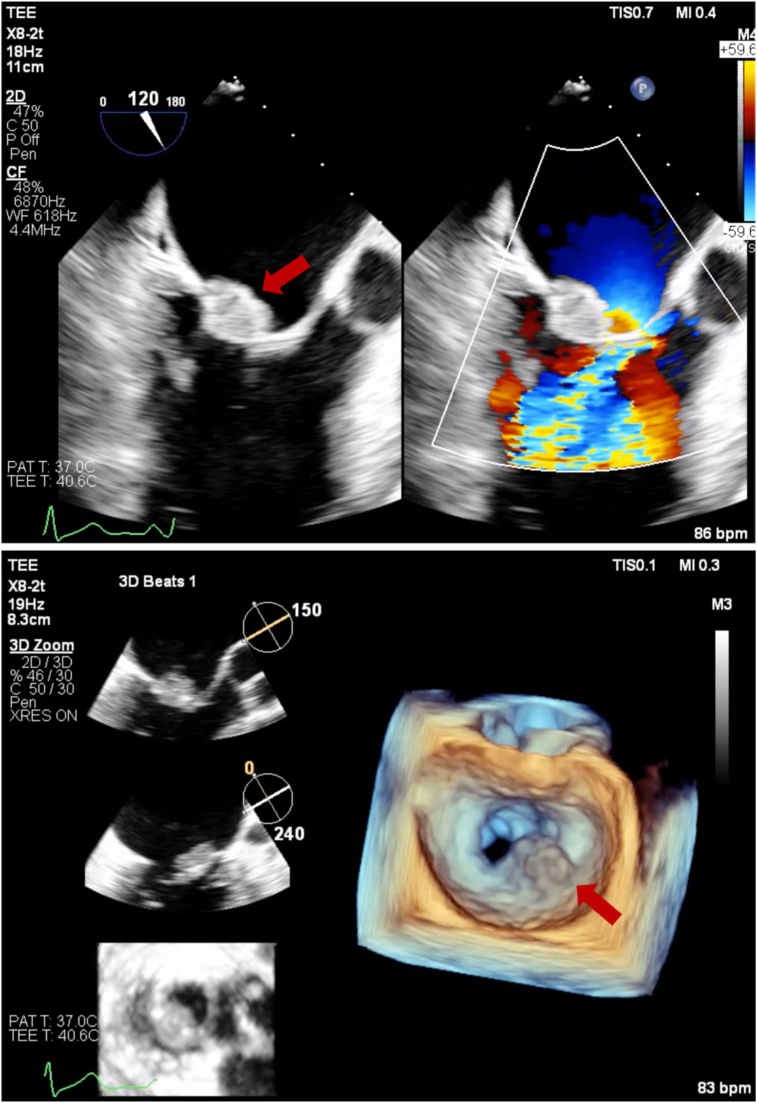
Two-dimensional TEE, mid-esophageal zoomed long-axis (120°) diastolic views (*top panel*) without (*left*) and with (*right*) color-flow Doppler, and 3D TEE (*bottom panel*) with multiplanar diastolic reconstruction displays in orthogonal long-axis orientation (*top, middle*) and short-axis orientation (*bottom*) and volume-rendered diastolic reconstruction (*right*), demonstrate a 1.9 × 1.8 cm vegetation localized to the P2 scallop of the PMVL (*arrows*) causing restricted opening and flow obstruction.
